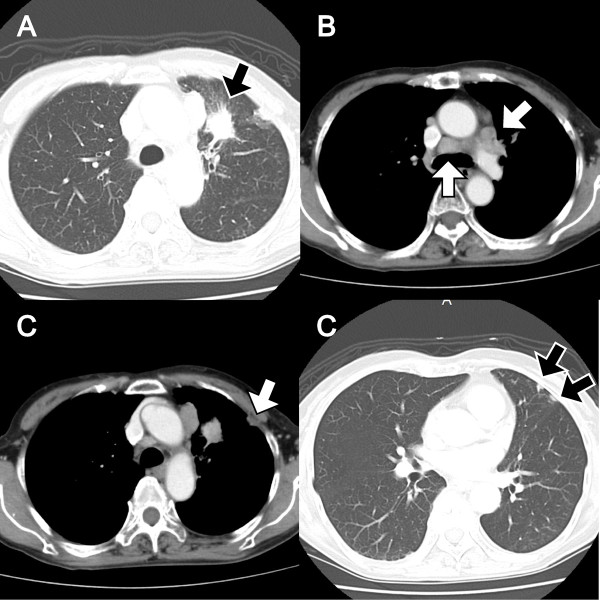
Computed tomography at the time of initial diagnosis. A) Primary lung cancer lesion in the upper lobe of the left lung. B) Enlargement of the mediastinal lymph nodes. C) Left pleural dissemination.
